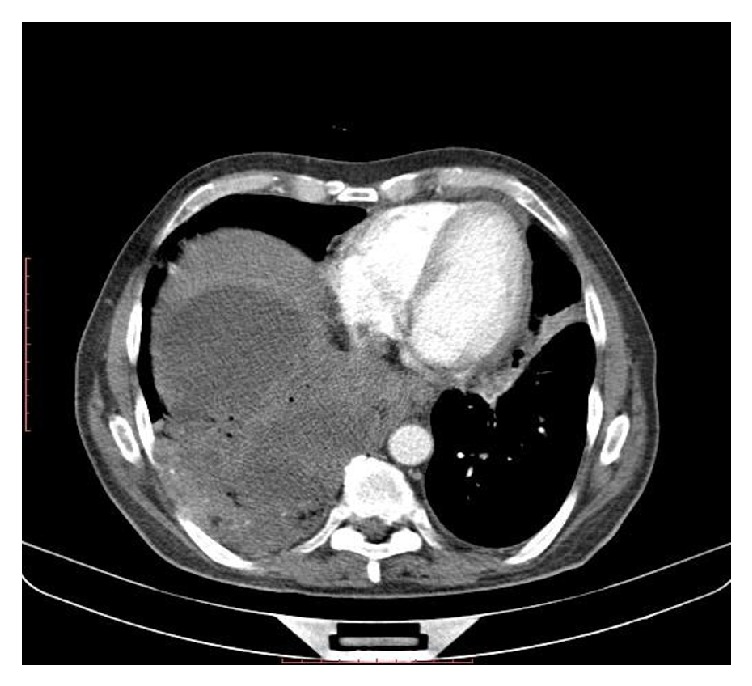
Ruptured hydatid cyst in lung via diaphragm on CT scan.
